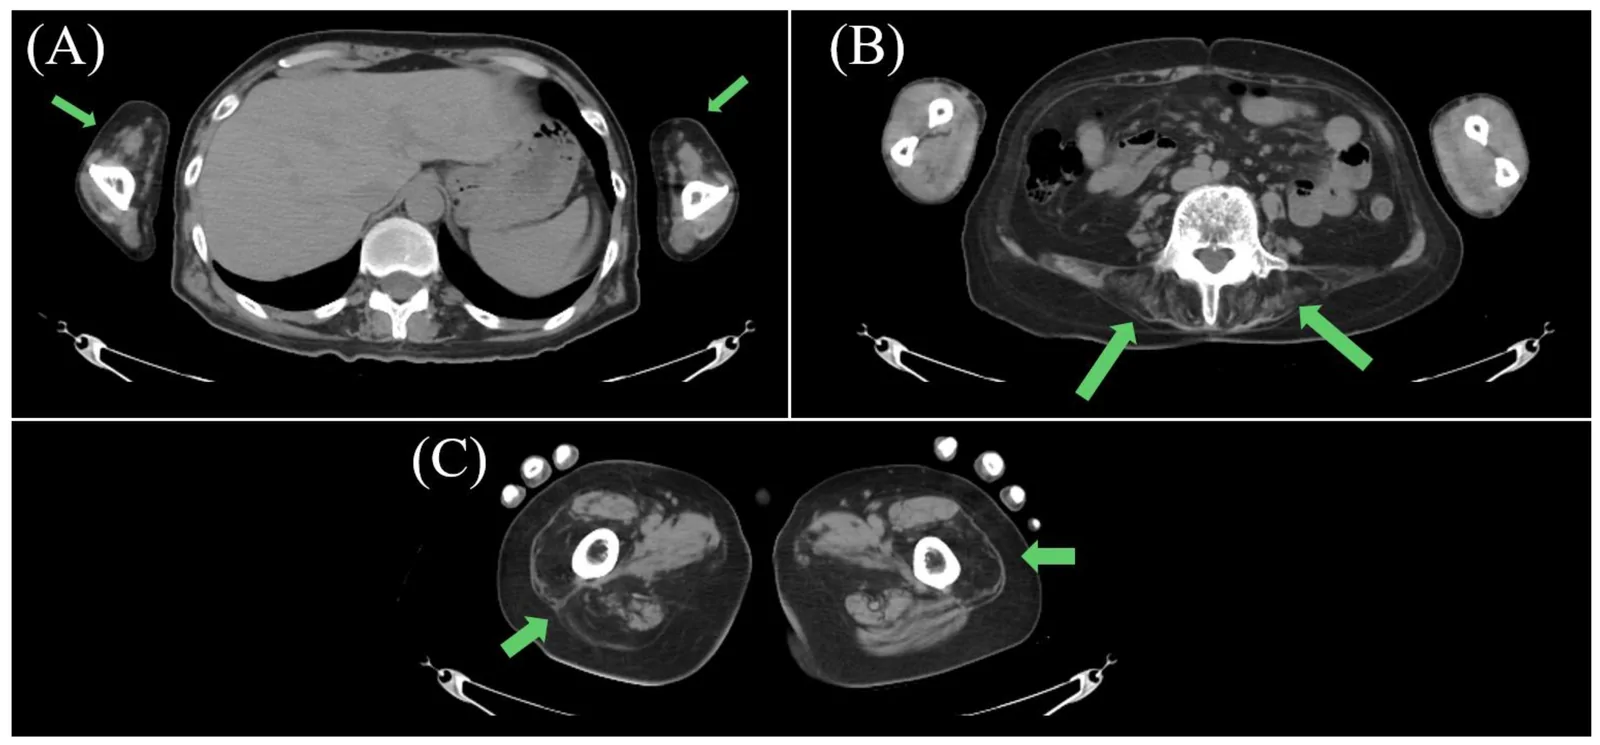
Muscle computed tomography (CT) findings. The CT scan showing muscle atrophy predominantly in the proximal muscles, including the upper arms (A), paraspinal muscles (B), and thighs (C) (green arrows).
